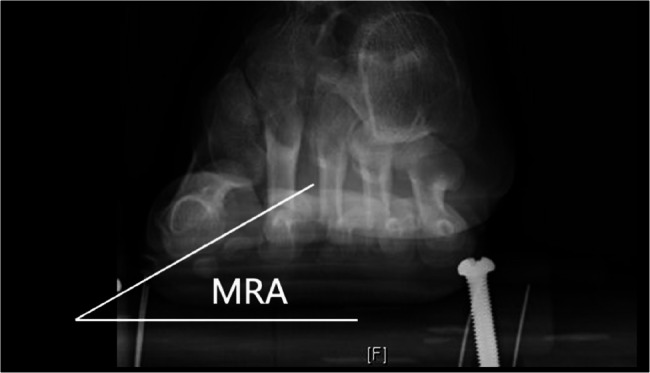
MRA in tangential sesamoid radiograph
